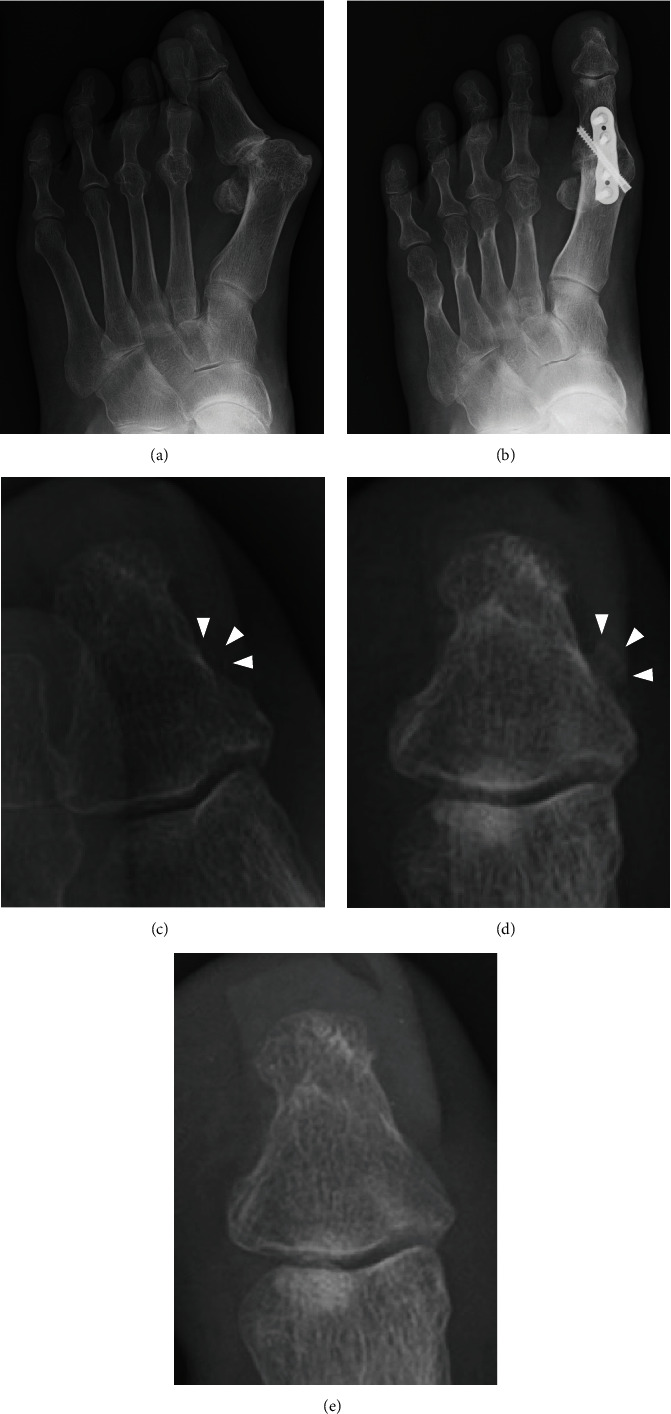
A case of symptomatic medial bone excrescence of the distal phalanx of the hallux after the metatarsophalangeal joint arthrodesis. Anteroposterior foot radiographs before surgery (a) and one year after surgery (b). Magnified images showing obscure excrescence before surgery (c), defined excrescence after arthrodesis (d), and disappeared excrescence after excision (e). Arrowheads indicate the bone excrescence.
